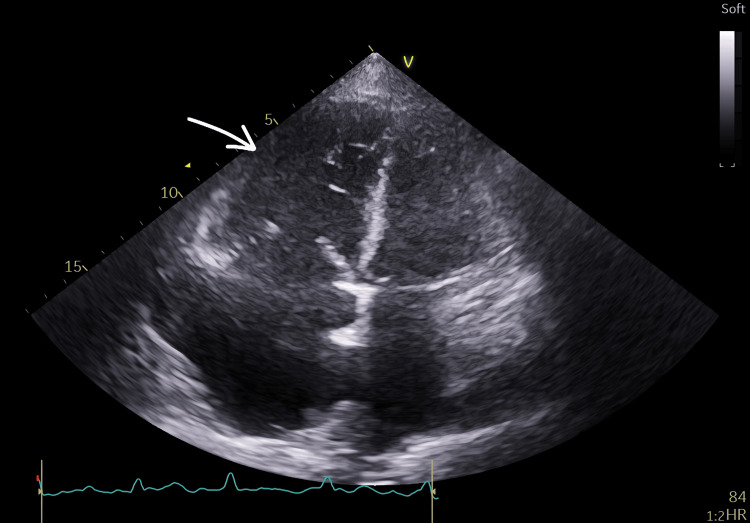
Echocardiogram Echocardiogram report included findings of severely dilated right ventricle (arrow) at the basal and mid-level with preserved basal contractility. Regarding normal left ventricle wall thickness, there was evidence of inter-ventricular septal flattening seen throughout the cardiac cycle, indicating increased right-sided pressure and volume overload. Thickened tricuspid valve with loss of leaflet apposition and severe tricuspid regirgitation noted, and dilated inferior vena cava and left atrium, all suggestive of pulmonary hypertension and right heart strain.
